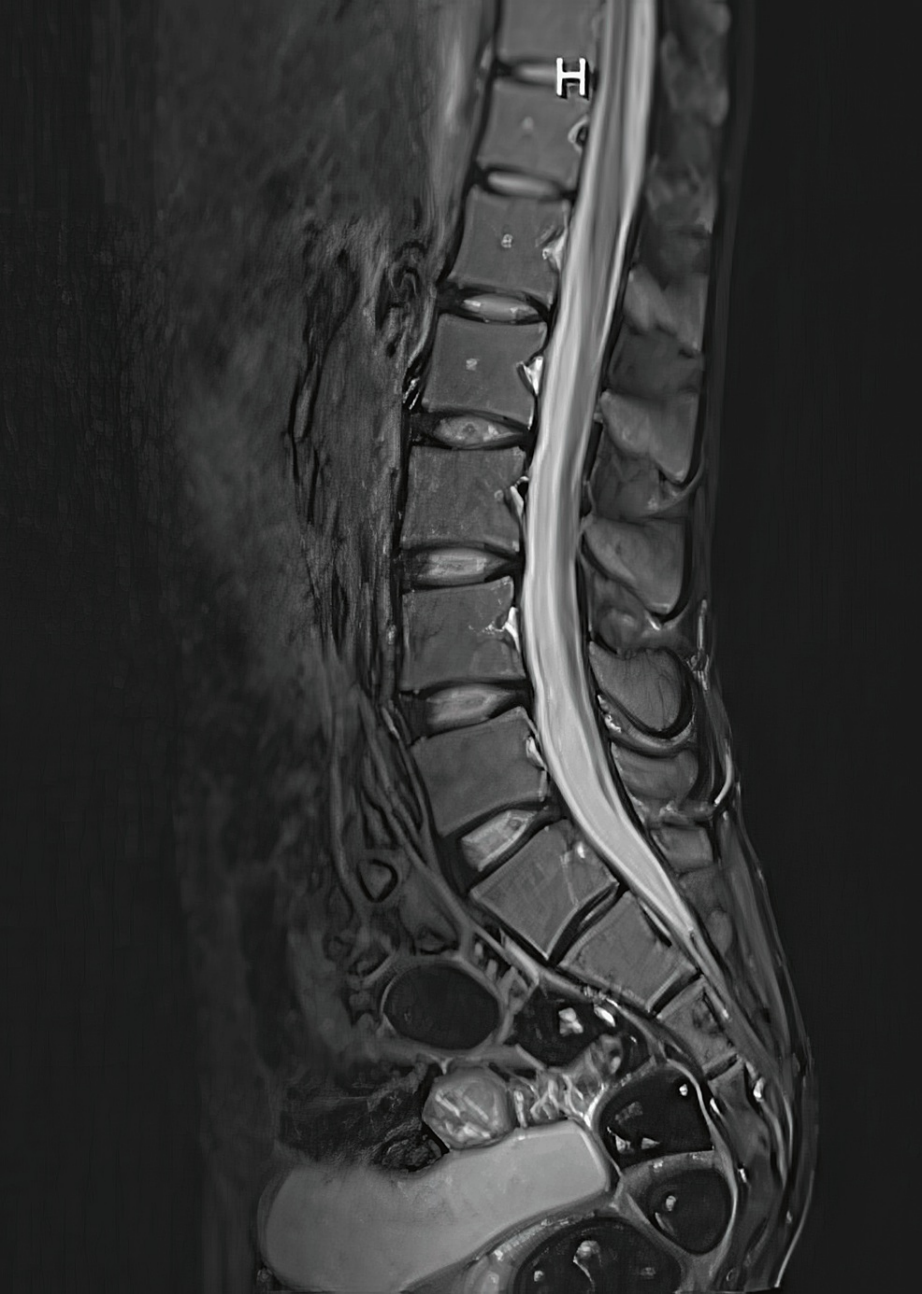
Normal MRI lumbar spine, T2 weighted image
